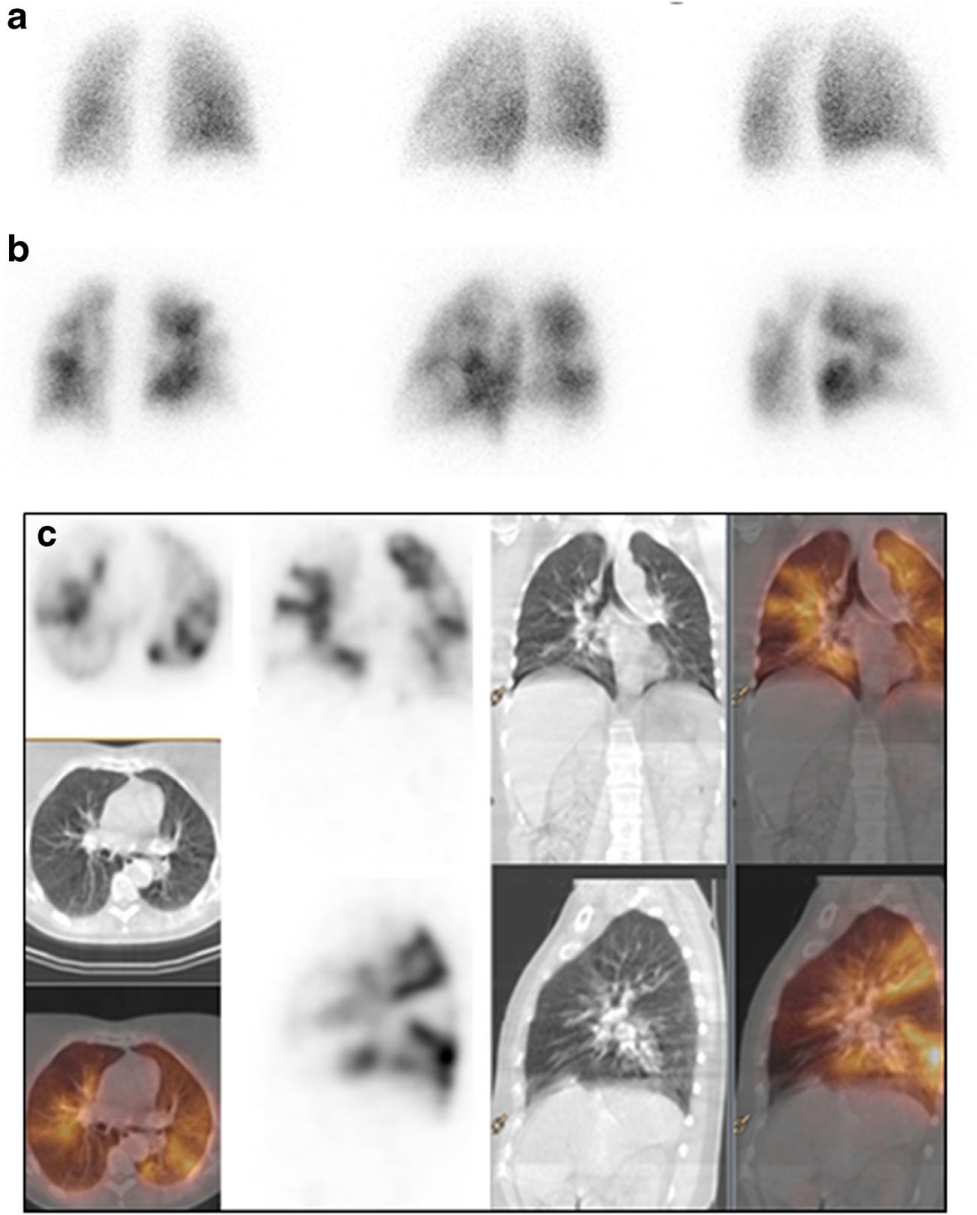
Lung SPECT/CT, perfusion study in suspected pulmonary embolism (PE). A 60-year-old patient with clinical suspicion of pulmonary embolism was referred for pulmonary ventilation/perfusion scintigraphy. Planar scintigraphy selected posterior and posterior oblique views) shows normal ventilation (a), in the presence of multiple segmental and subsegmental perfusion defects in both lungs (b). SPECT/CT shows no corresponding abnormal parenchymal changes on the CT component of the study (c). The patient was diagnosed with a bilateral pulmonary embolism
